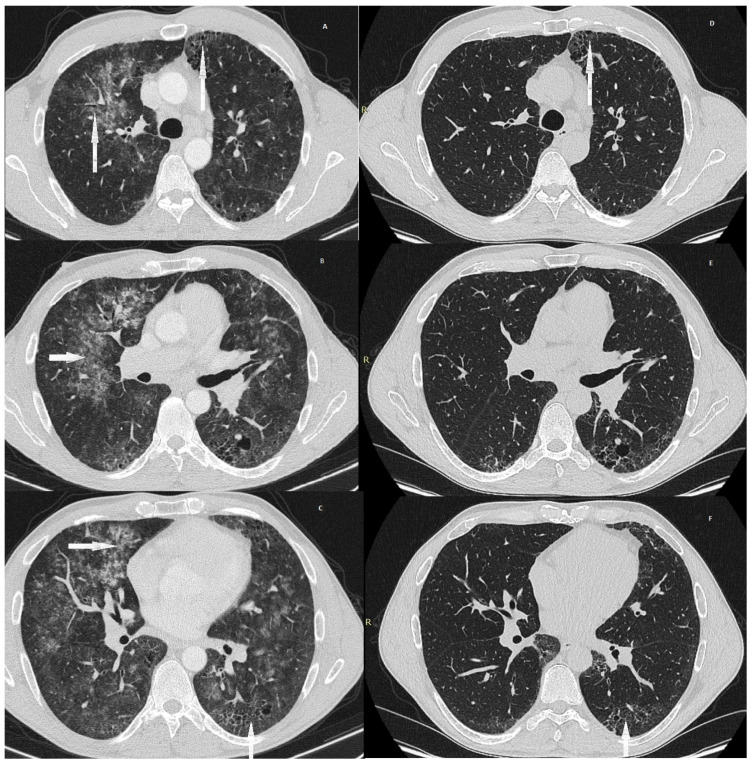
(A–C): High-resolution computed tomography (HRCT) during a recurrent episode of diffuse alveolar haemorrhage (DAH). Diffuse bilateral ground-glass opacities are present, superimposed on pre-existing reticulation. Arrows highlight areas of fresh ground-glass opacities consistent with acute alveolar haemorrhage, underlying reticulation reflecting chronic interstitial lung disease and small foci of honeycombing where visible. (D–F): Follow-up HRCT demonstrating complete resolution of acute alveolar infiltrates. Persistent reticulation and traction bronchiectasis remain, consistent with fibrotic remodelling. Arrows indicate residual reticulation and traction bronchiectasis.
